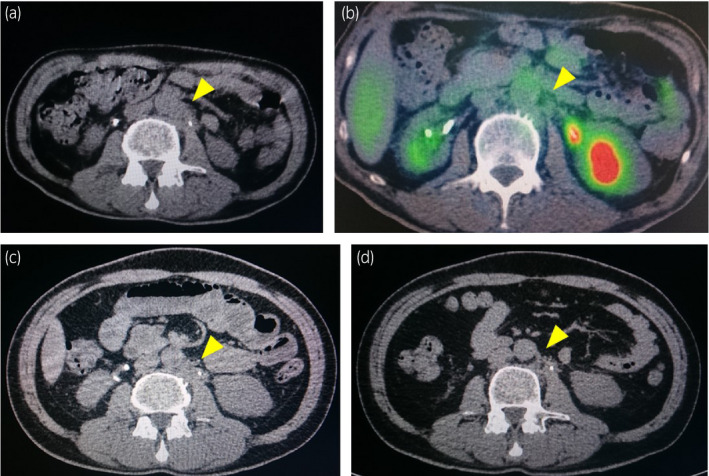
Findings from postoperative abdominal CT and FDG PET/CT. (a, b) Abdominal CT and FDG PET/CT show para‐aortic lymph node metastasis (yellow arrow) appearing 7 months after cystectomy. Increased accumulation (SUVmax 2.4, yellow arrow) is seen in the lesion on FDG PET/CT. (c) After 5 cycles of pembrolizumab therapy, the metastatic lesion (yellow arrow) had decreased in size. (d) After 10 cycles, the lesion remained undetectable on CT (yellow arrow). We evaluated the complete response by RECIST version 1.1.
